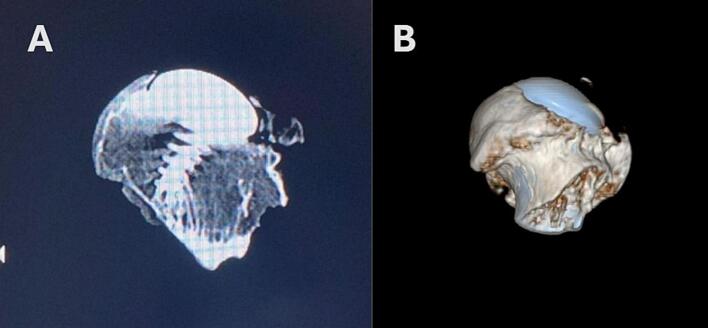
Coronal (A) and volume rendering reformatted (B) CT images demonstrate the integration of resurfacing impant with the bone and the absence of loosening or fracture.
